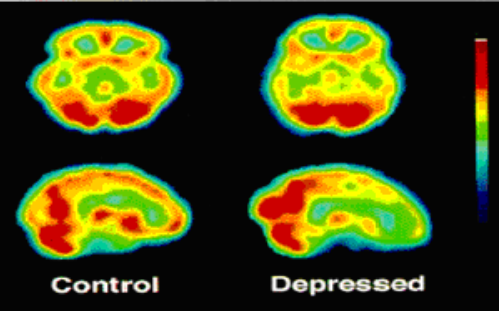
Single photon emission computed tomography (SPECT) images from a depressed patient showing characteristic hypofrontality relative to a healthy control subject[82].
